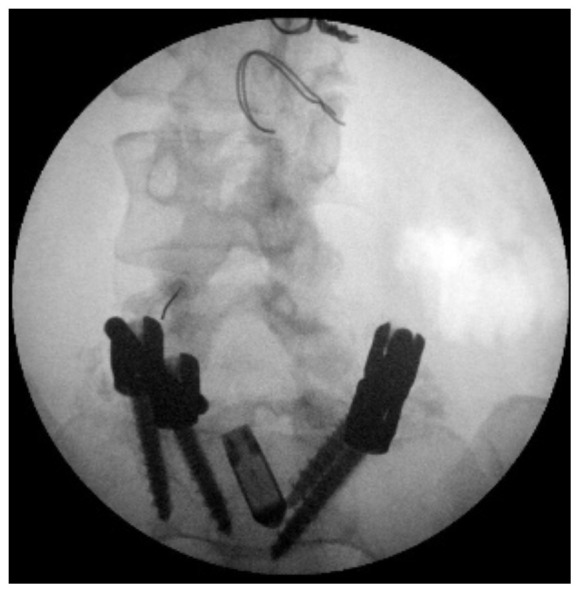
X-ray image of the L4-L5 facet joints before implantation.
